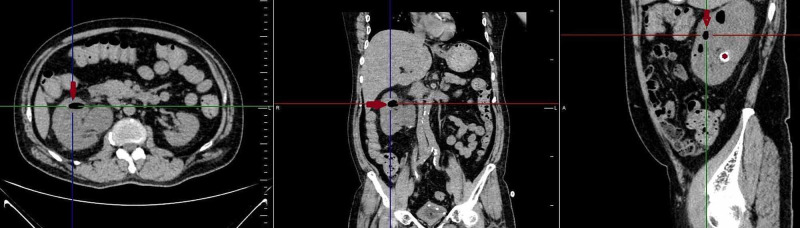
Reformatted plain CT abdomen in axial, coronal, and sagittal sections revealed air in the renal pelvis and calyces (red arrows). A large radiodense calculus (red asterisk) was seen towards the inter-polar region on the sagittal section.
